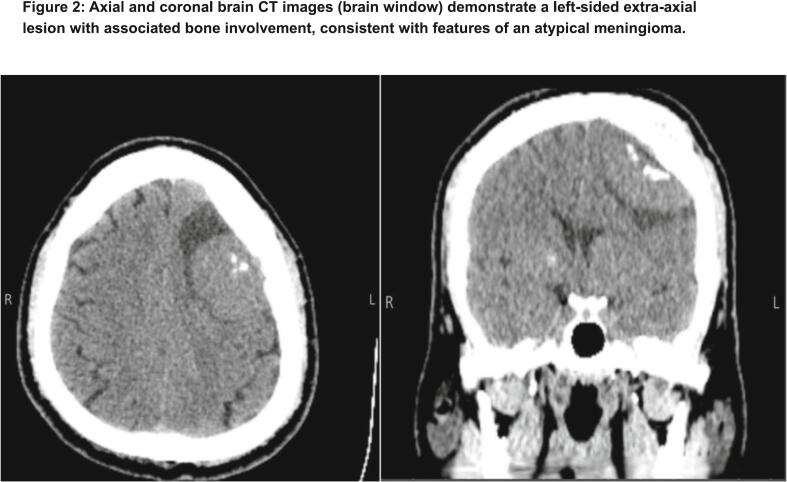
Axial and coronal brain CT images (brain window) demonstrate a left-sided extra-axial lesion with associated bone involvement, consistent with features of an atypical meningioma.
